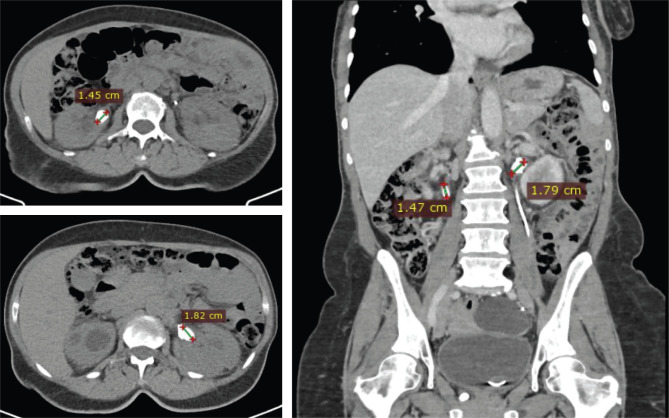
CT sections measuring different dimensions of bilateral renal stones of the same case.
